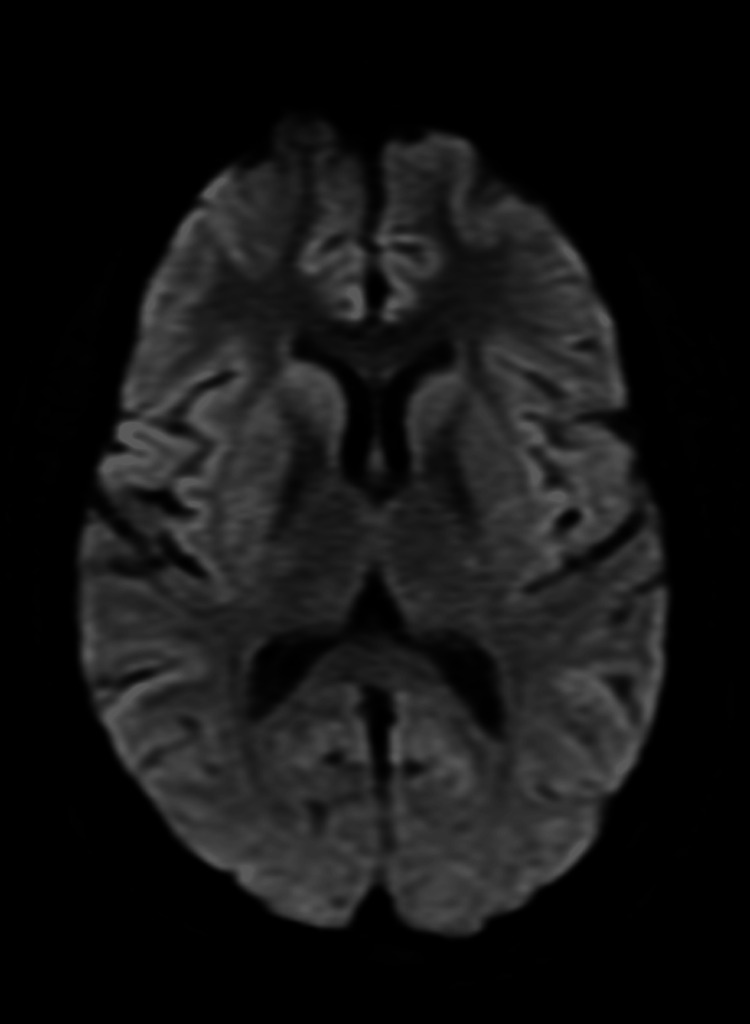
MRI brain (DWI) showing no restricted diffusion in the basal ganglia and occipital region. MRI: magnetic resonance imaging; DWI: diffusion-weighted imaging
